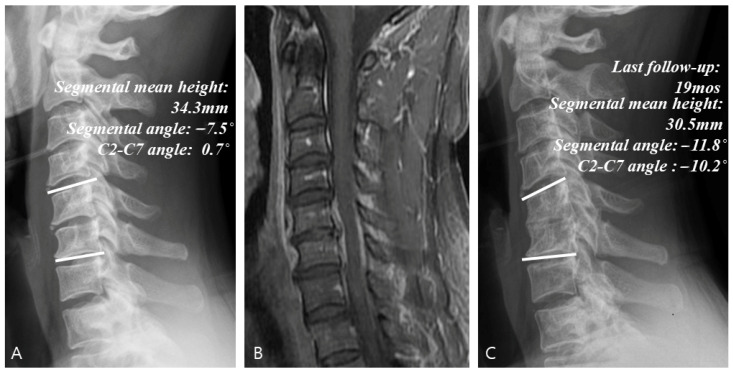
Example of a case of conservative treatment. (A) Simple radiographs of a 51-year-old male patient showing C5–C6 disk space narrowing and osteolytic change. (B) Gadolinium-enhanced MR image showing infectious spondylodiscitis at C5–C6, accompanied by prevertebral and epidural abscess. (C) Last follow-up (19 months) plain radiographs showing worsening radiologic parameters at C5–C6.
